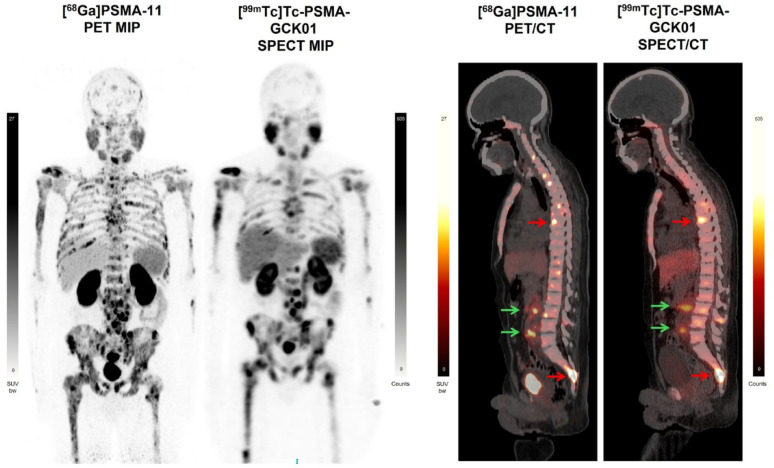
A 61-year-old patient with disseminated bone metastases in advanced stage of prostate carcinoma underwent both PSMA PET/CT and SPECT/CT scans for determination of phenotyping prior to RLT. Red arrows indicate bone metastases; green arrows indicate lymph node metastases
